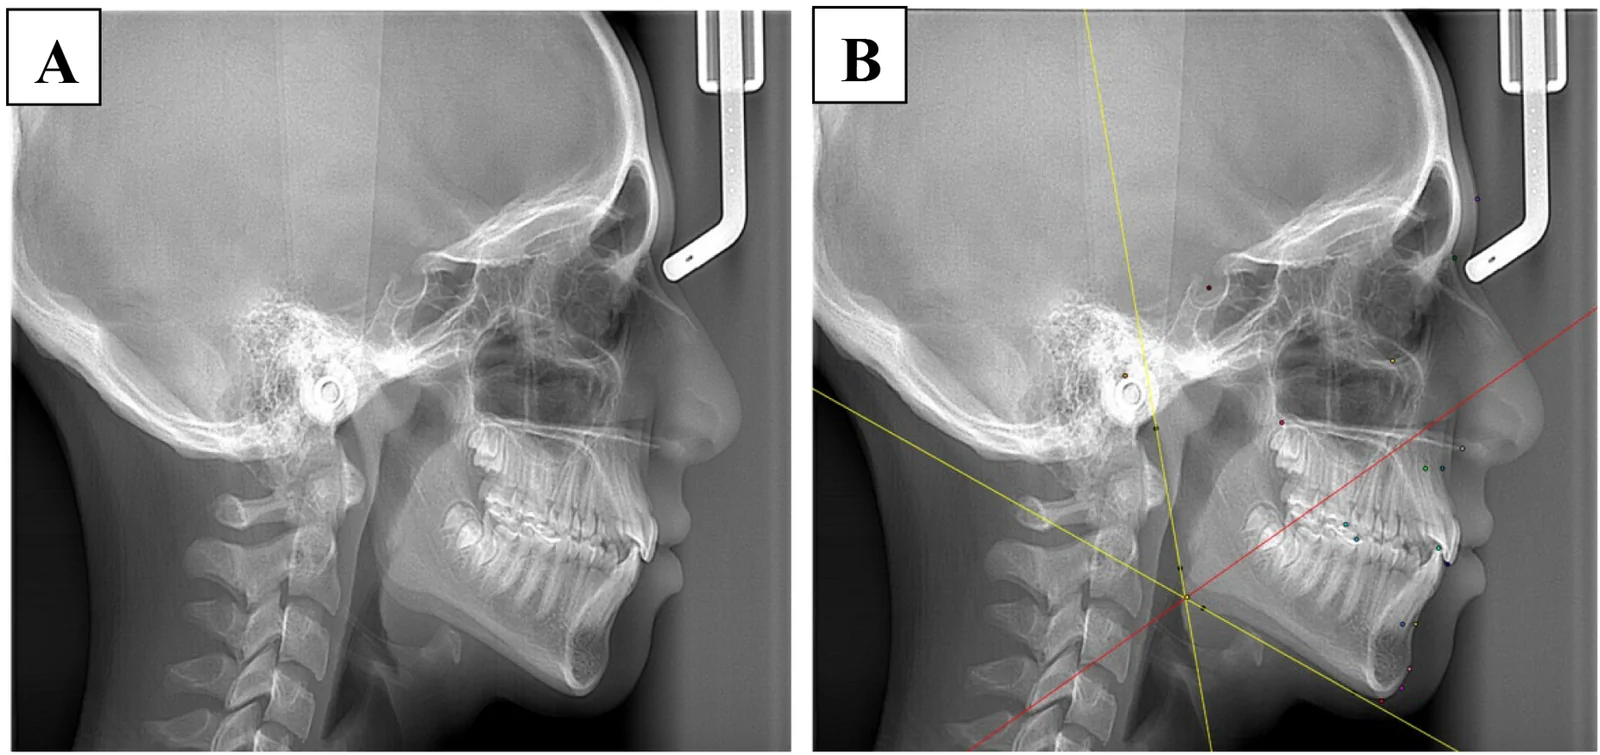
(A) Lateral cephalometric x-ray. (B) AI training to identify the points in the cephalometric training phase.
